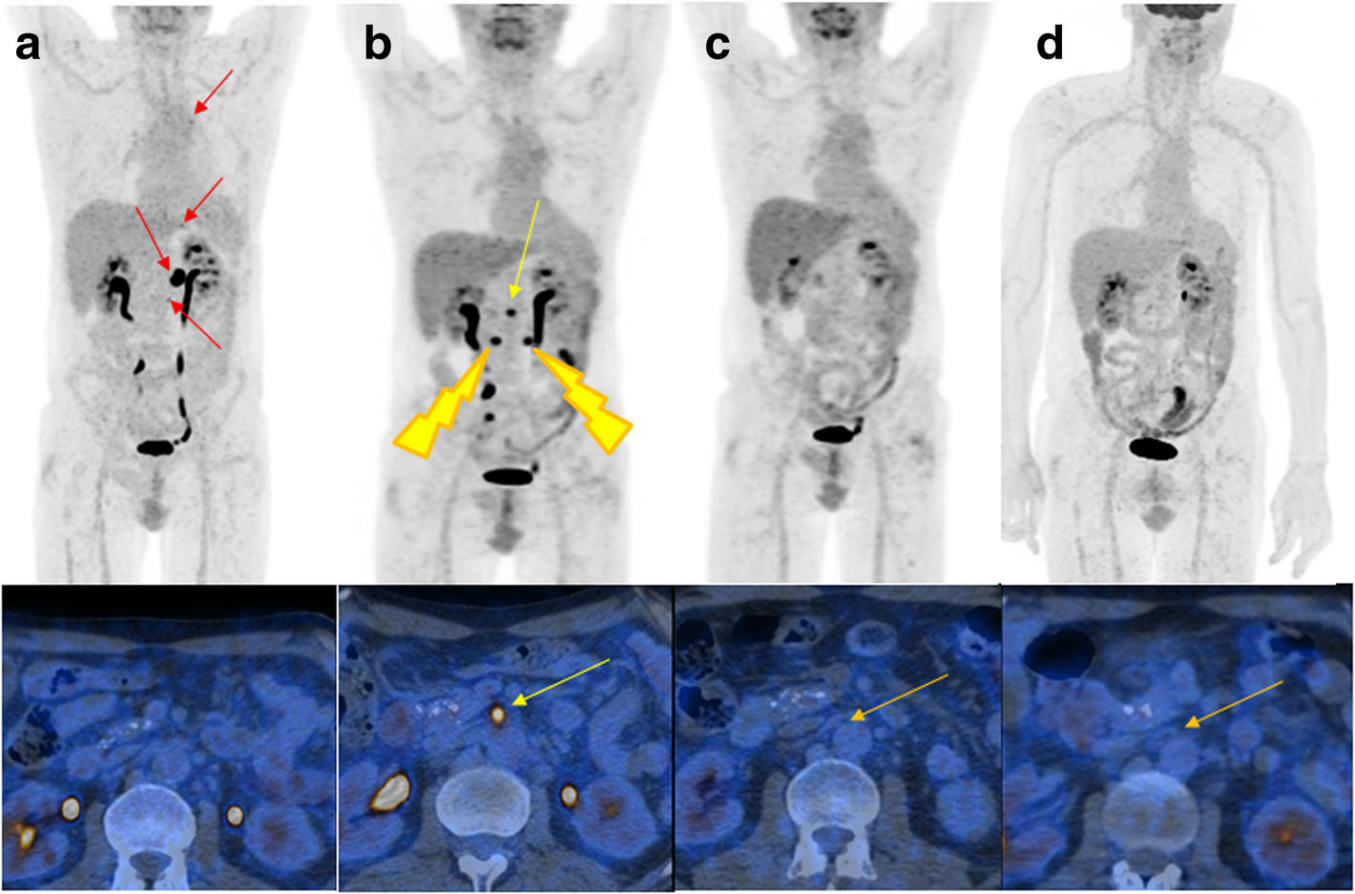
a PET/CT staging before start of treatment with nivolumab: the red arrows indicate the localization of the lymph node metastases. b PET/CT re-staging after 6 cycles of nivolumab with evidence of complete response of the previous metastases and appearance of new metastases, indicated by yellow arrows. Two out of the three new metastases were irradiated, as indicated. c PET/CT restaging 10 weeks after radiotherapy with evidence of complete response. d PET/CT restaging two years after start of nivolumab confirming a stable complete remission
